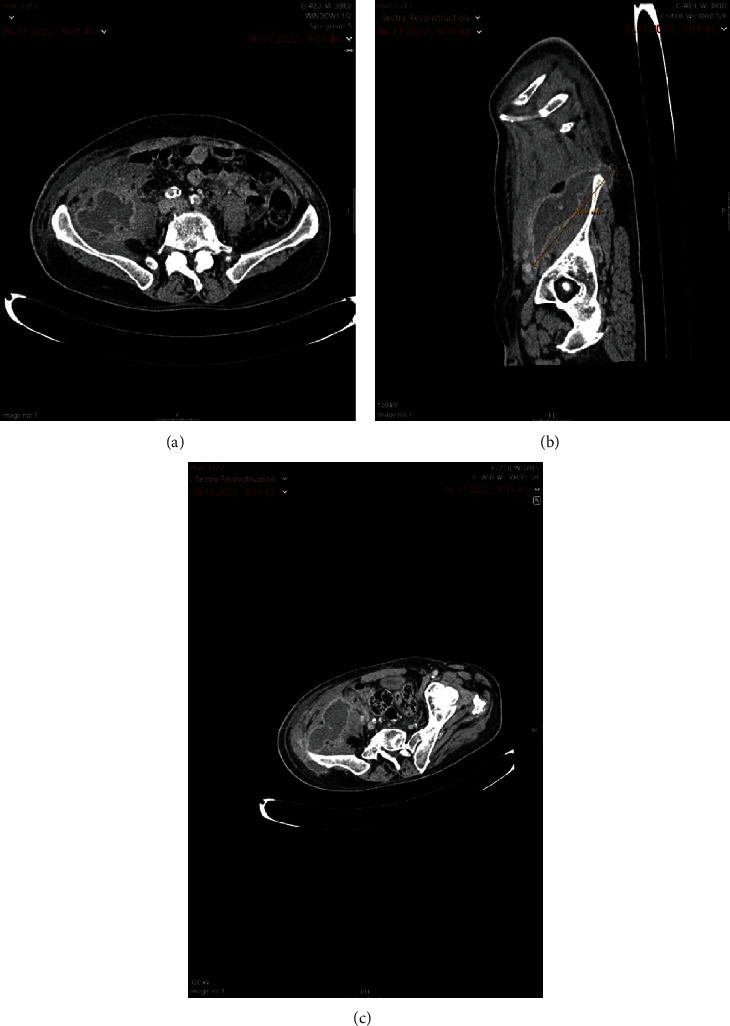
(a–c) Contrast-enhanced computed tomography (CECT) of abdomen and pelvis showing multiobulated, well-marked, and encapsulated dense fluid collection, spreading from pelvic retroperitoneal space to posterior pararenal space and subcutaneous soft tissue above right iliac crest. The collection also spreads into the right iliopsoas and oblique abdominal muscle.
